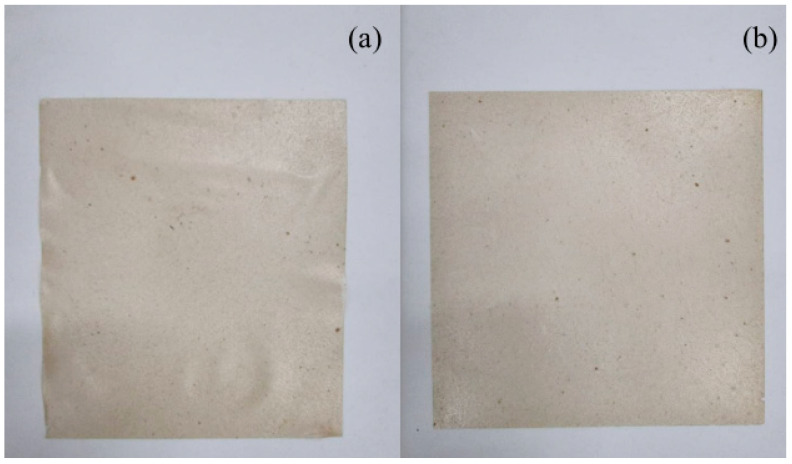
Photographs of film fabricated using (a) control starch and (b) ultrasound-extracted starch.
